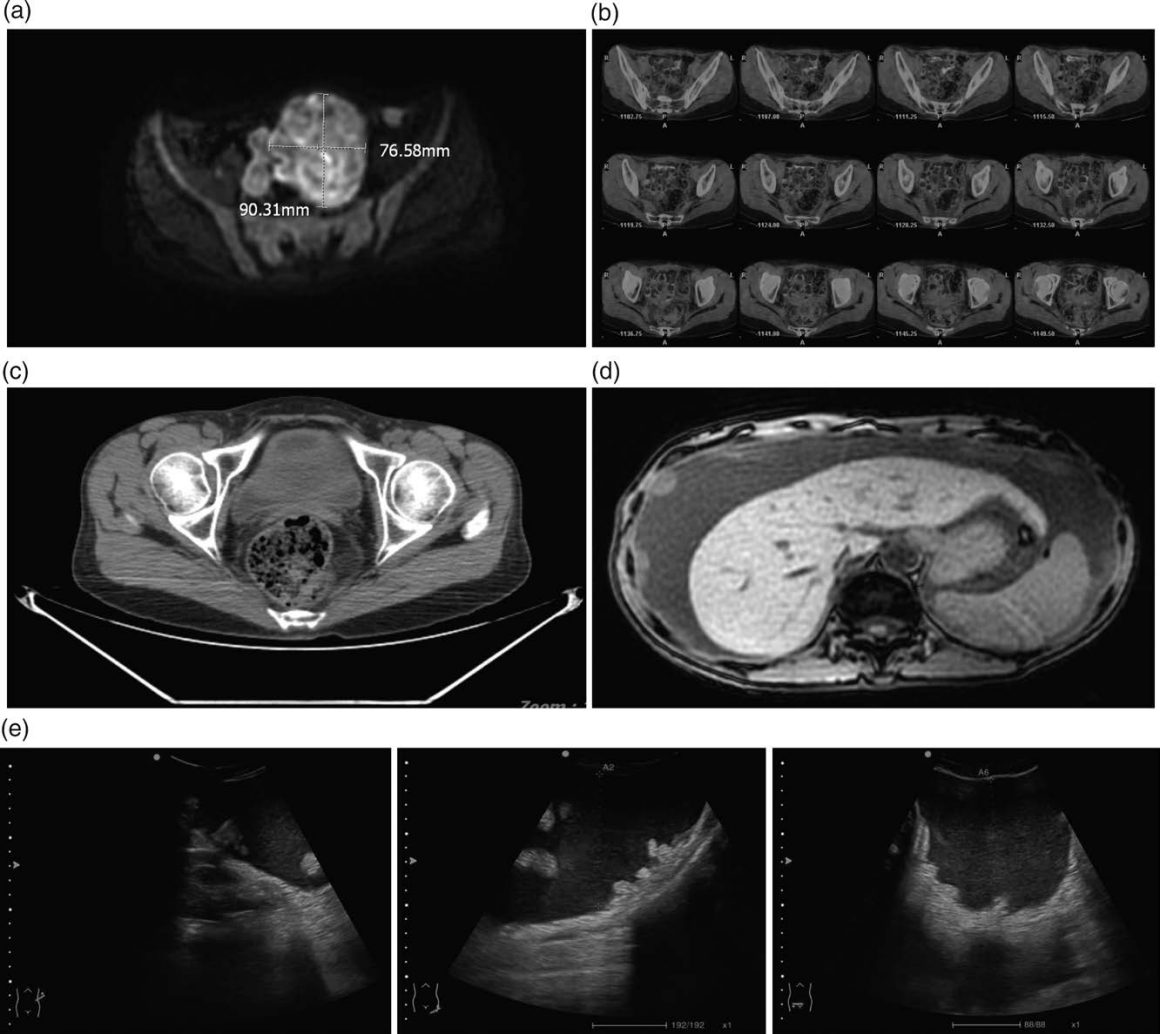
Findings for imaging examinations. (a) Abdominal MRI findings. (b) Abdominal PET-CT. (c) Representative images of CT plain scan. (d) Abdominal CT findings after relapse. (e) Abdominal ultrasound after relapse. CT, computed tomographic.
